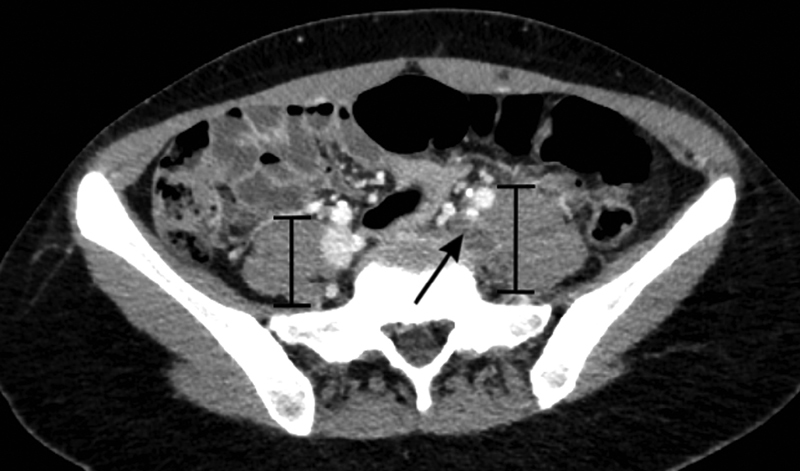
Postcontrast axial view of CT of abdomen/pelvis demonstrating a clot within the left common iliac vein (
